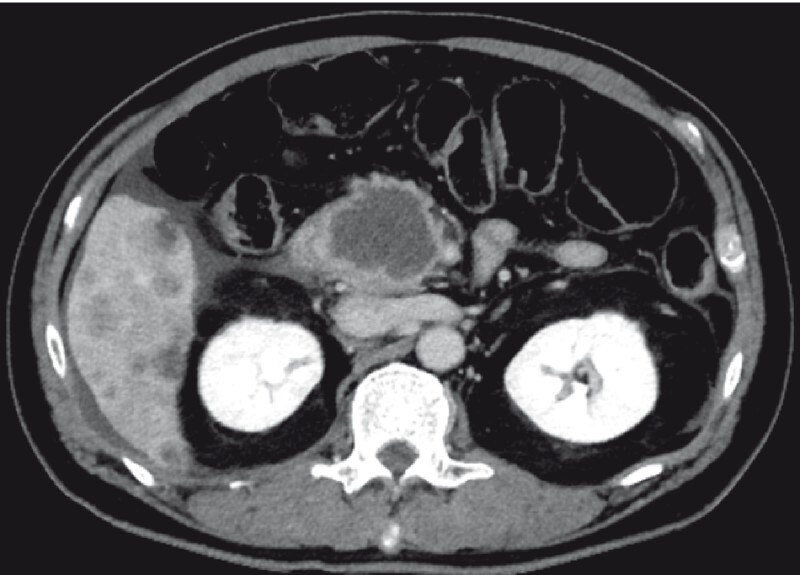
Case 3: Contrast-enhanced computed tomography image indicated a hypovascular pancreatic head tumor, 50 mm in diameter, with multiple liver metastases. It was suspected that the inside of the tumor was necrotic.
